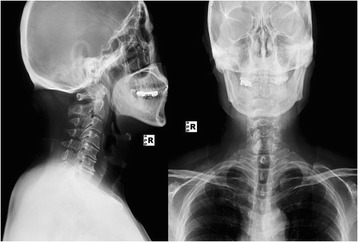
Postoperative x-ray showing laminoplasty with suture fixation keeping the lifted lamina open
